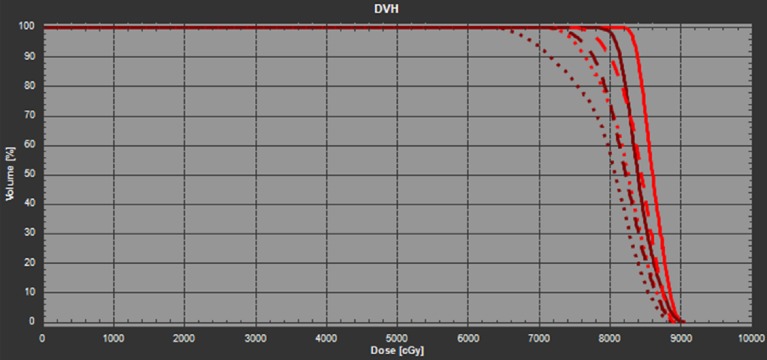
The DVH of the nominal plan in the planning CT (solid line), +3 degree (CW) rotated CT (dashed line), and -3 degree (CCW) rotated CT (dotted line). The red lines denote the iGTV and the dark brown lines denote the CTV. CCW: Counter-clockwise; CT: Computed tomography; CTV: Clinical target volume; CW: Clockwise; DVH: Dose volume histogram; iGTV: internal gross target volume.
